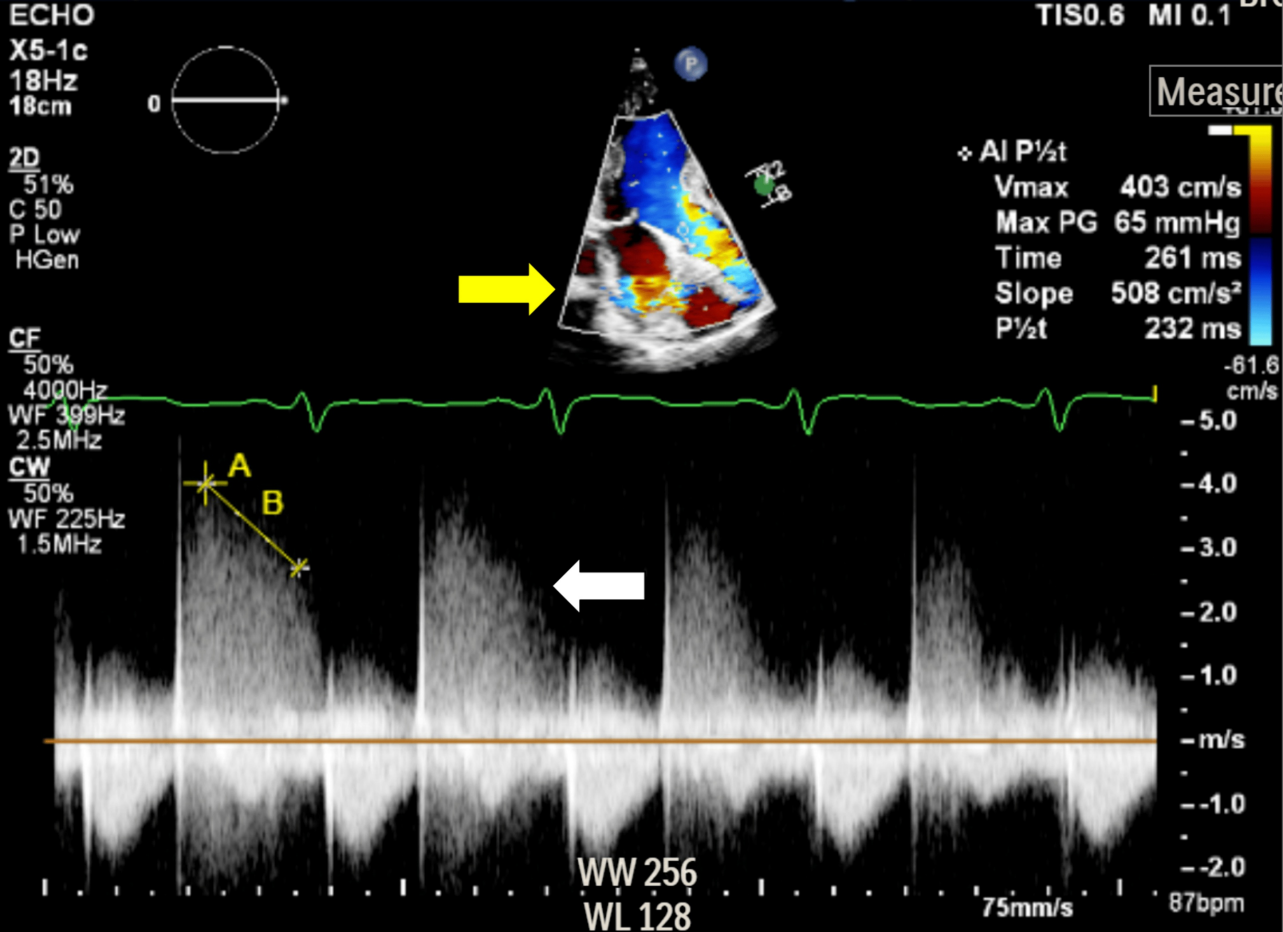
Measurement of moderate-to-severe aortic regurgitation using color flow (yellow arrow) and continuous-wave Doppler (white arrow).
